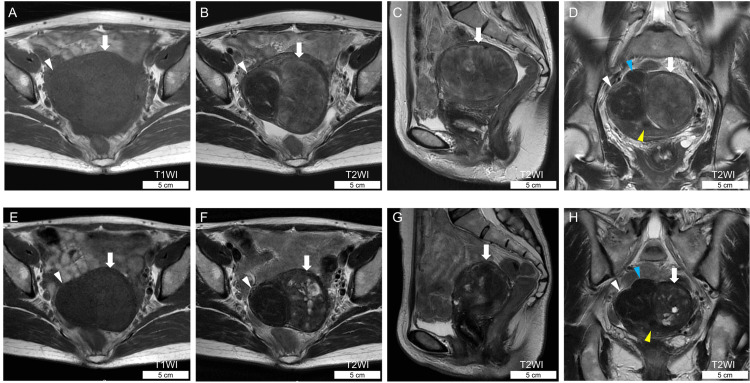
Magnetic resonance images of the uterine IMT. (A,B,E,F) Horizontal sections. (C,G) Sagittal sections. (D,H) Coronal sections. Scale bars are included in the images (A-D) MR images obtained before GnRH antagonist therapy. The uterine IMT (indicated by arrows) exhibits isointensity on T1WIs (A) and heterogeneous signal intensity on T2WI (B-D). The second-largest mass (leiomyoma) (indicated by white arrowheads), the third mass (indicated by blue arrowhead), and the fourth mass (indicated by yellow arrowhead) demonstrate low intensity on T1- (A) and T2- (B-D) WIs. (E-H) MR images obtained four months after the initiation of GnRH antagonist therapy. The uterine IMT (indicated by arrows) appears reduced in size and shows increased hyperintensity on T2WI (F-H) compared to pretreatment images. The second-largest leiomyoma (indicated by white arrowheads) appears slightly decreased in size. The other two leiomyomas (indicated by blue and yellow arrowheads) appear unchanged MR: magnetic resonance; GnRH: gonadotropin-releasing hormone; IMT: inflammatory myofibroblastic tumor; WI: weighted image
